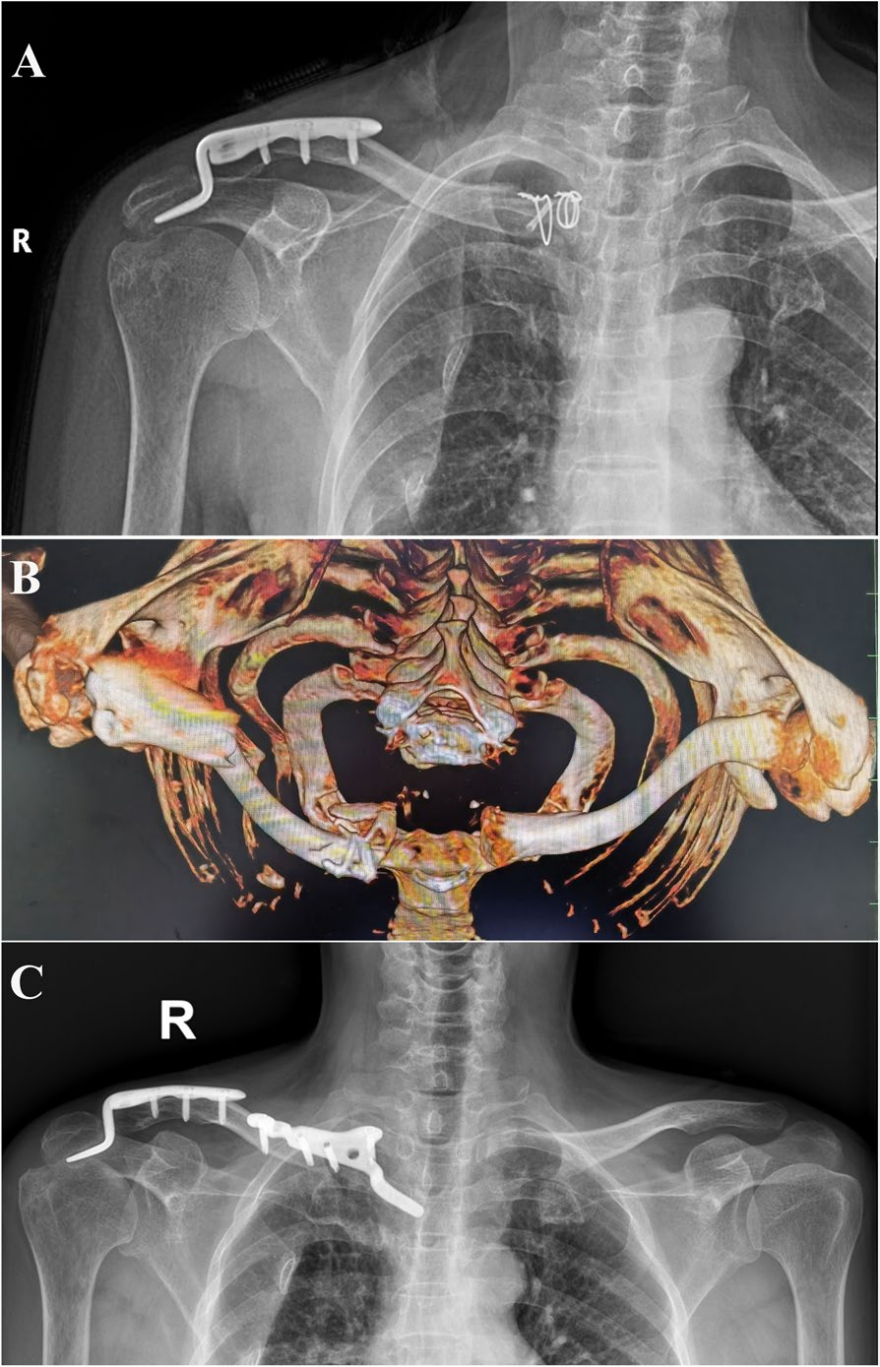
A 62-year-old woman (Patient 5) underwent both medial and distal end fixation in local hospital. She experienced continuous medial clavicle protrusion and pain, and a (A) posteroanterior radiograph and (B) three-dimensional computed tomography reconstruction from above taken 1 month after surgery showed re-displacement of the medial clavicle bone fragments. She underwent a revision operation involving removal of the initial implant and fixation with a clavicle hook plate. C At the 6-month follow-up, the patient showed radiographic congruency of both the sternoclavicular joint and acromioclavicular joint
